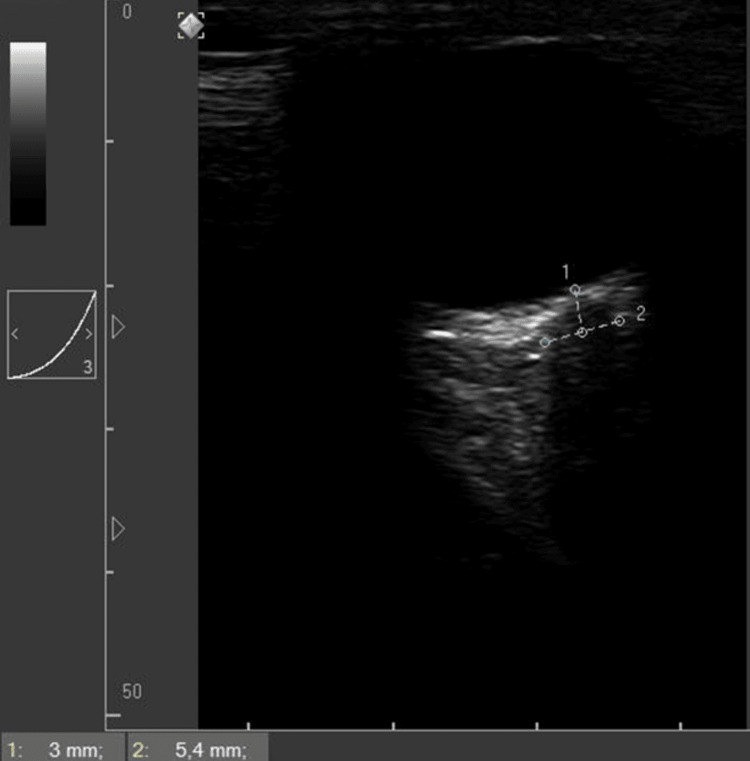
Ocular ultrasonography. (1) Optic disc height (ODH): 3.0 mm (Reference: < 0.5 mm), showing significant protrusion indicative of papilledema. (2) Optic nerve sheath diameter (ONSD): 5.4 mm (Reference: 4.6–5.0 mm), reflecting increased intracranial pressure. Measurements were obtained 3 mm posterior to the globe. Blood pressure at measurement: 125/80 (95)
